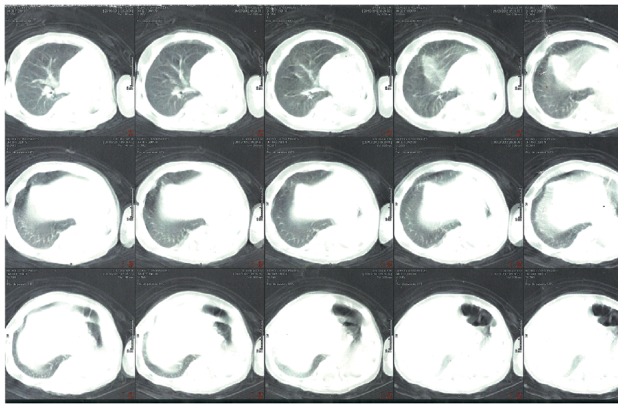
Chest CT scans showing absence of the left lung.
